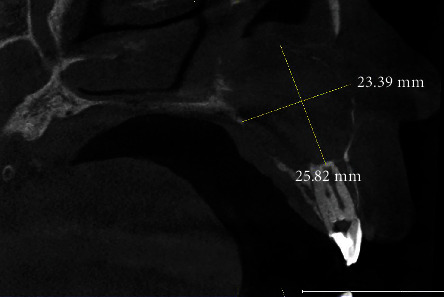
CBCT sagittal section confirmed that it is a type II DI, showing a wide radiolucent periapical image measuring 25.82 mm × 23.39 mm communicating with the nasal cavity.
